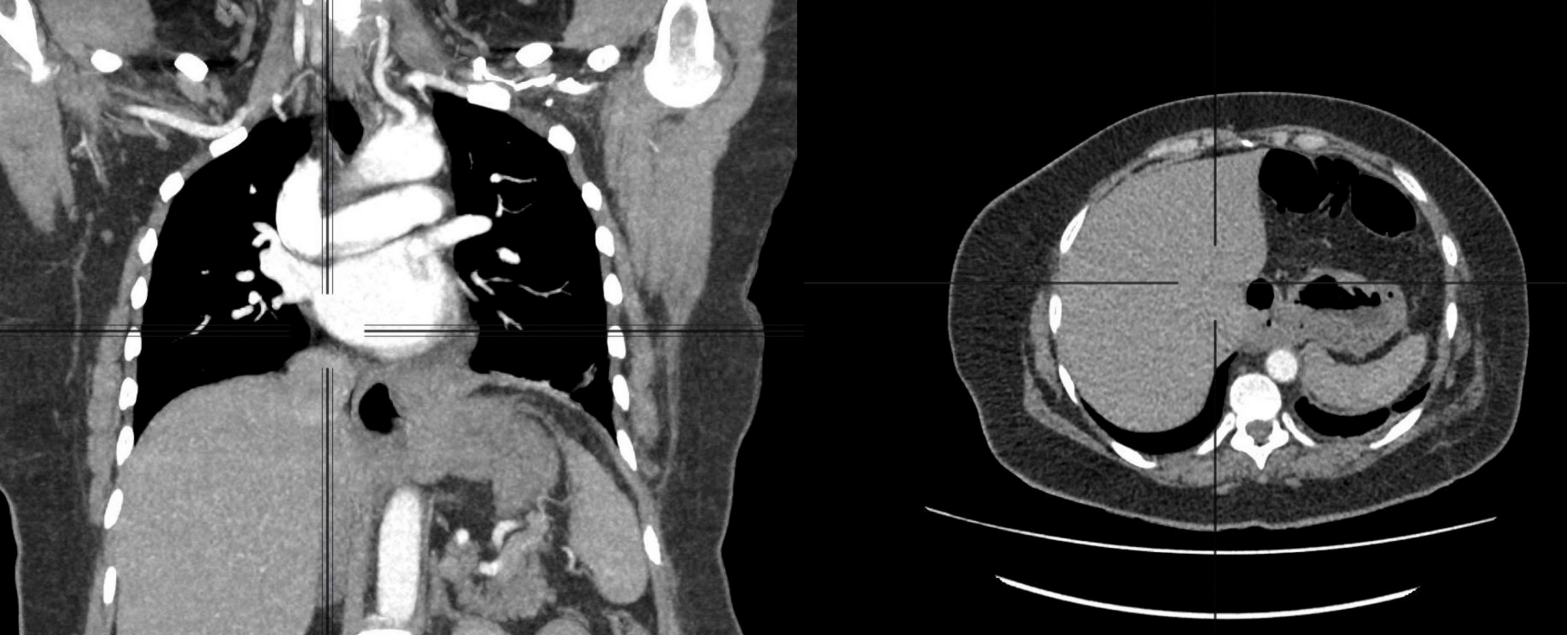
Control computed tomography, performed four months postoperatively, shows no evidence of recurrence or other abnormalities
